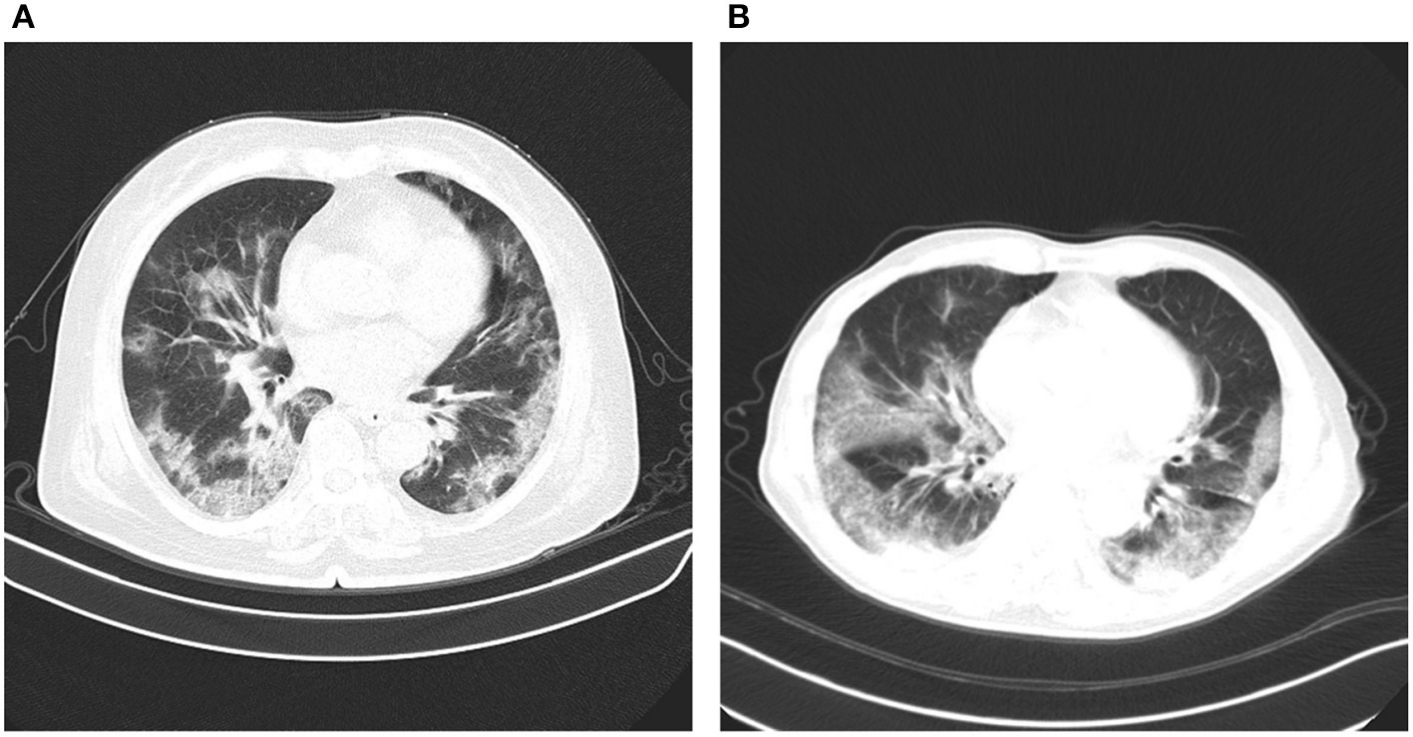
Example chest CT abnormalities in (A) a patient with COVID-19 pneumonia, and (B) a patient with non-COVID-19 pneumonia. It can be observed that visual differences in abnormalities between COVID-19 pneumonia and non-COVID-19 penumonia can be quite subtle.
